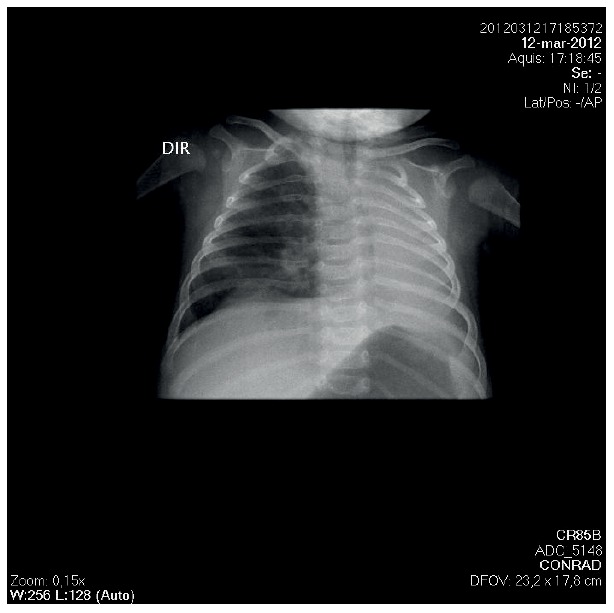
Chest X-ray showing absence of the left lung.
